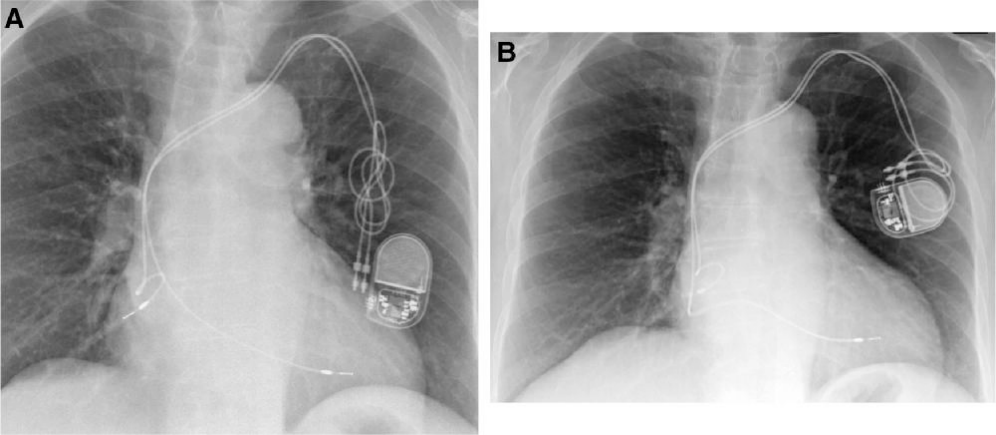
Case report 2. (*A*) Chest X-ray—3 months after the revision of pacing system. (*B*) Control chest X-ray—1 month after submuscular (pectoralis major muscle) implantation.
